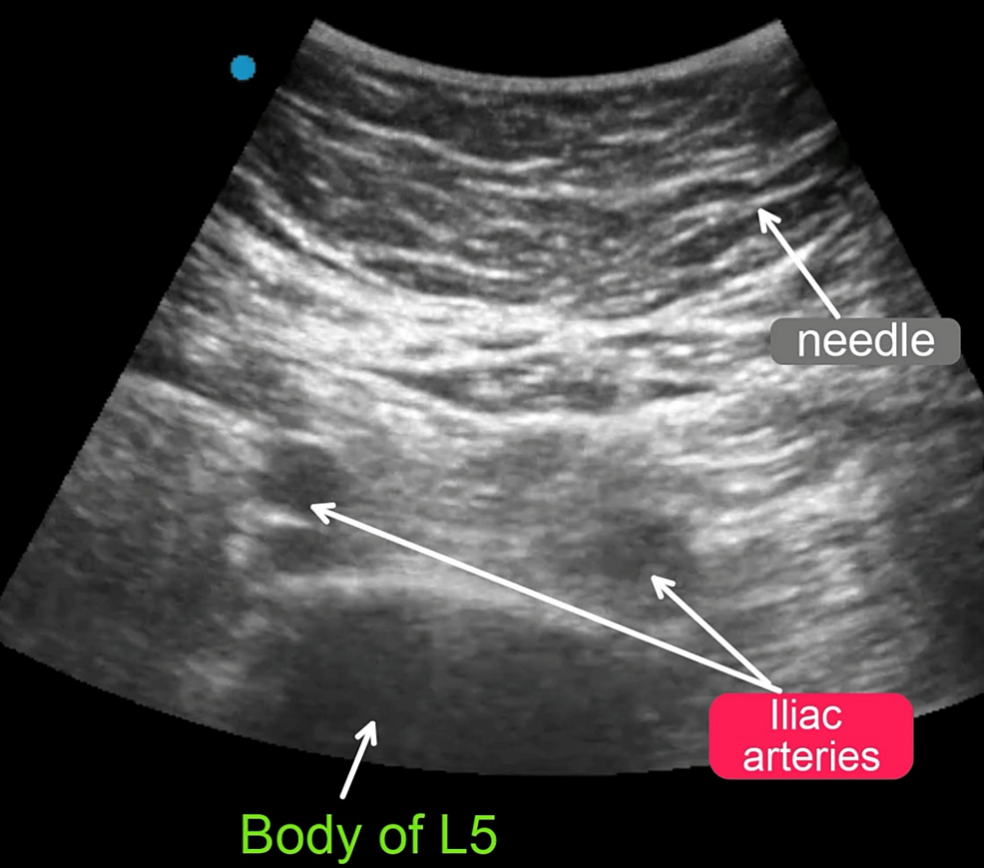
Ultrasonography displaying the location of the superior hypogastric plexus block
